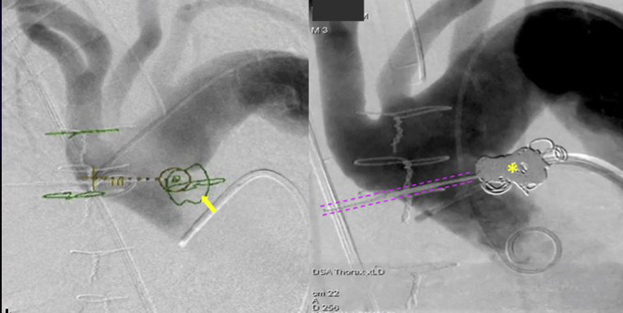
Percutaneous, transthoracic coil-embolization of an ascending aortic pseudoaneurysm.
